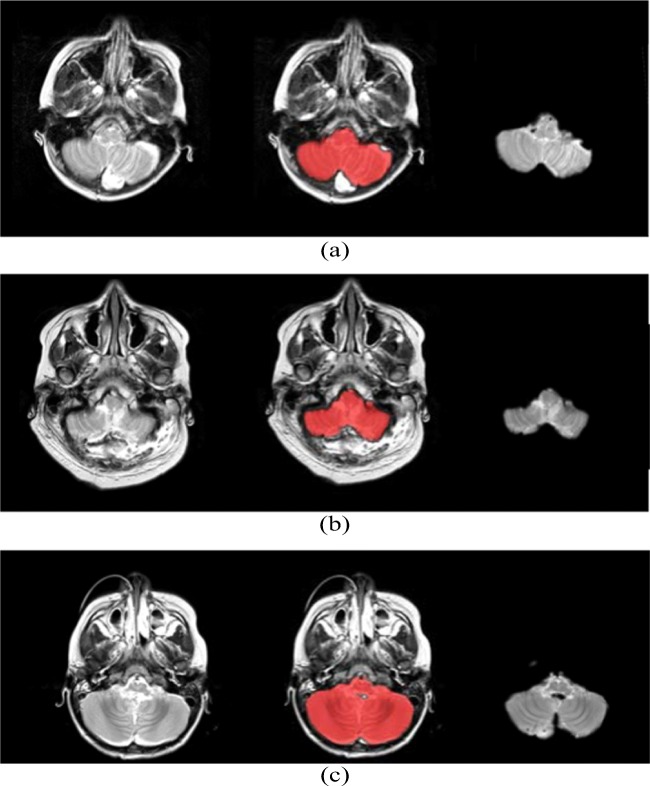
From left to right: original MR image slice, segmentation probability map, and isolated cerebellum on an arbitrary slice from a volumetric MR image for a Patient 9 b Patient 11 and c Patient 13
